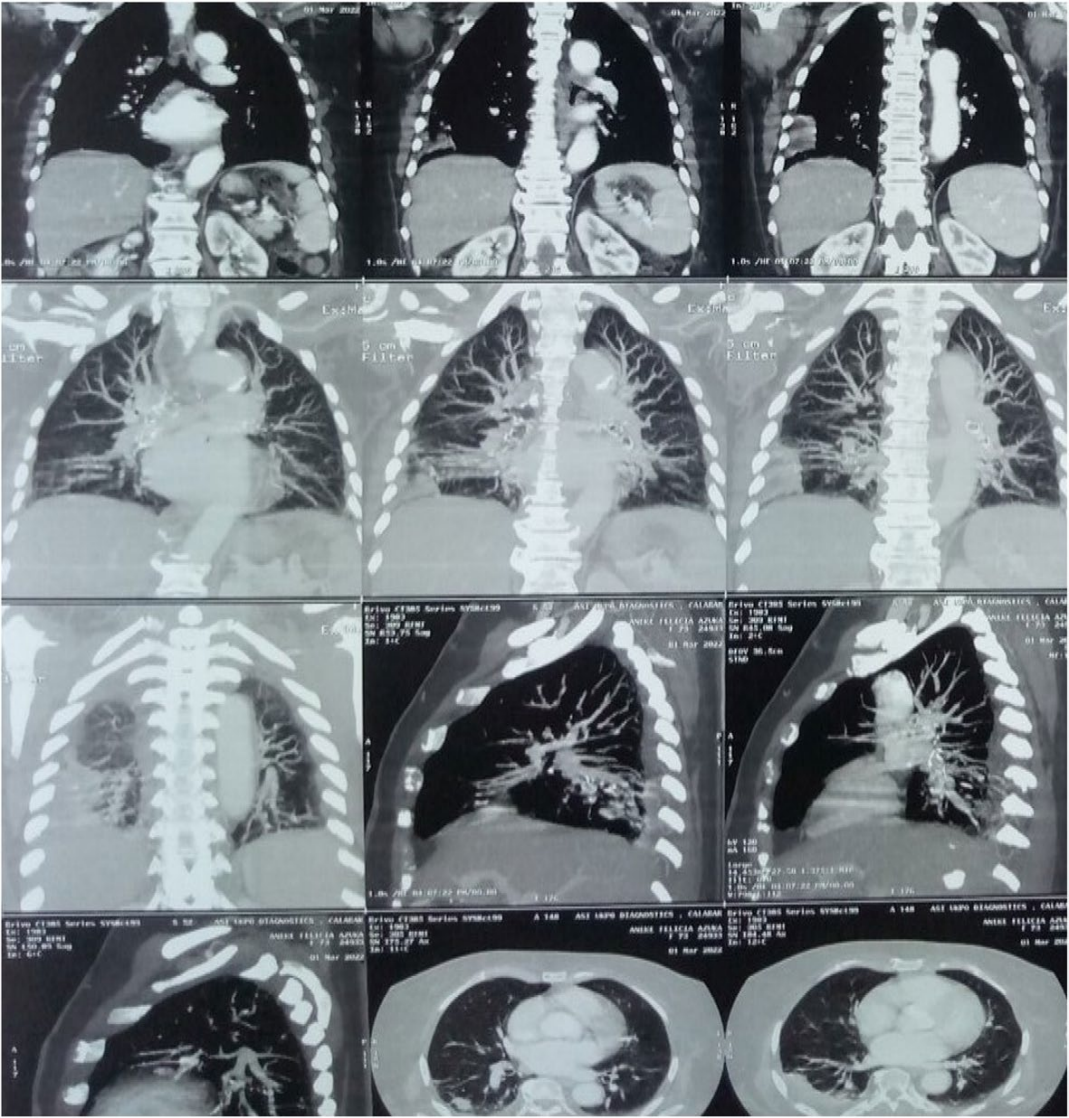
HRCT chest (contrast-enhanced) showing filling defects within the pulmonary arteries at the segmental and sub-segmental levels, pulmonary plethora, and right lower lobar consolidations
